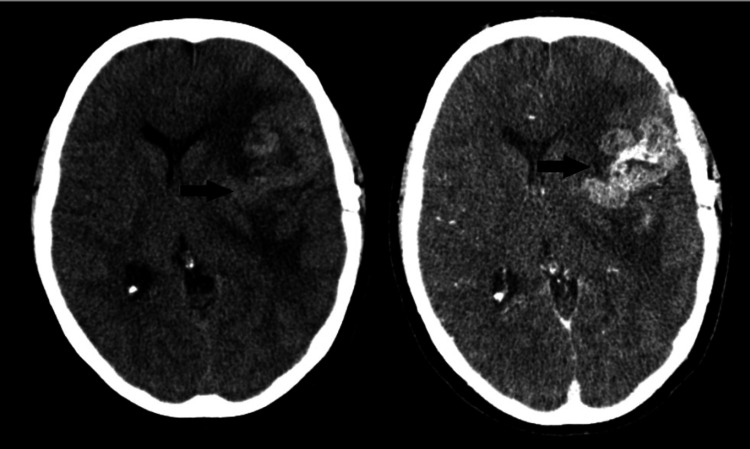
An unenhanced and enhanced CT scan of the brain demonstrates post-left parietal approach craniotomy changes with an enhanced hyperdense mass centered over the left insular cortex (black arrows).
